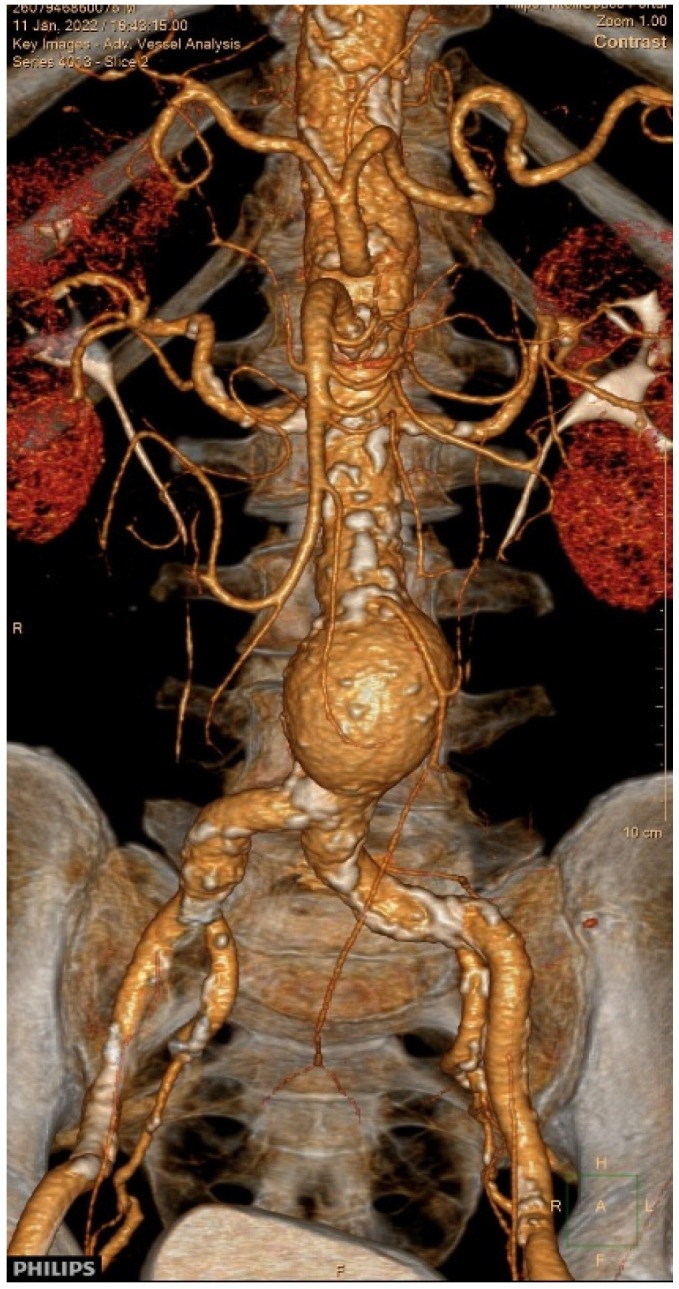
Three-dimensional computed tomography reconstruction of the abdominal aortic aneurysm.
